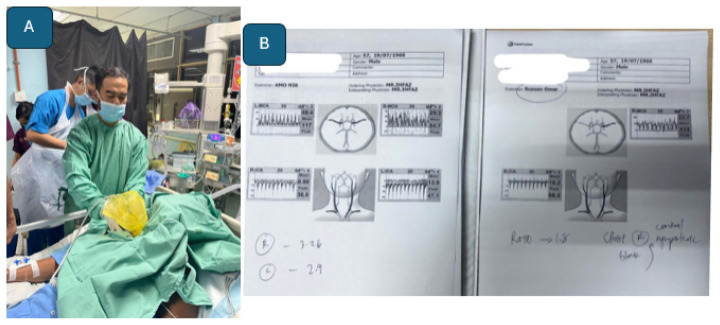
A) Stellate ganglion block performed under sterile technique to treat cerebral vasospasm; B) Transcranial doppler to measure cerebral blood flow pre- and post-stellate ganglion block (immediate response)
